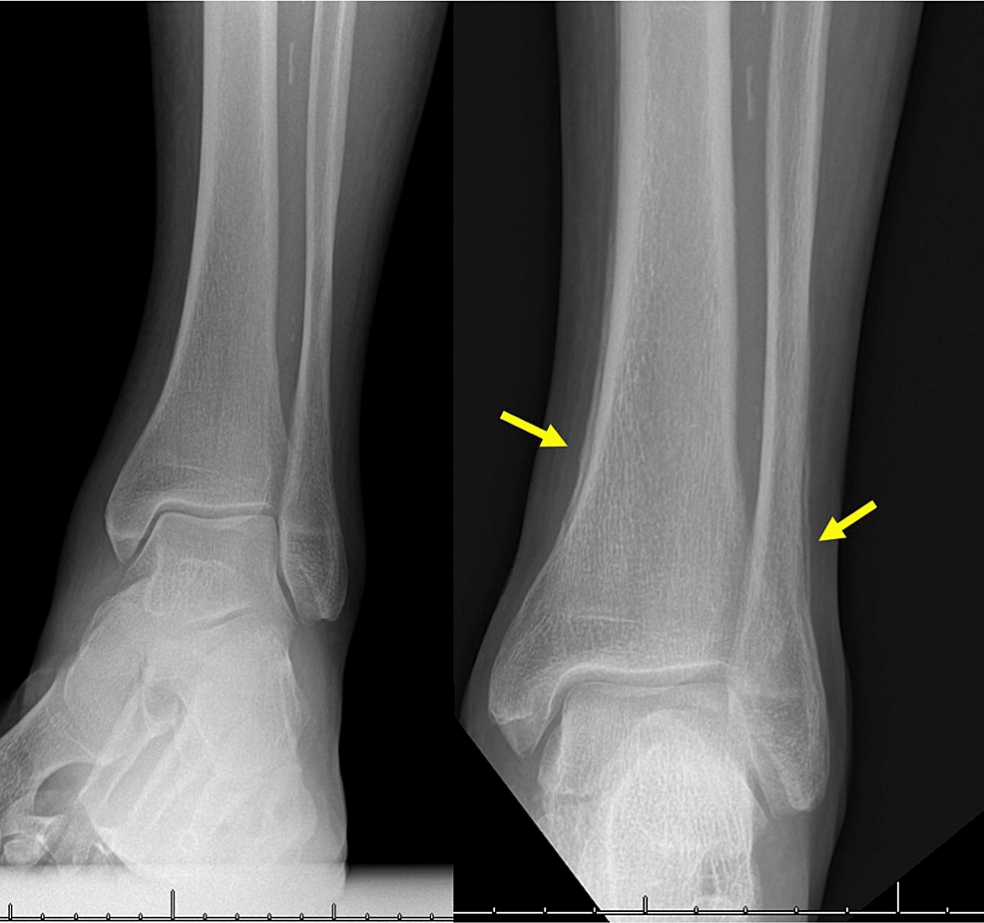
Radiological osteoarticular manifestations of the Pierre-Marie Bamberger syndrome X-ray of the left ankle in five-month intervals demonstrating the appearance of hypertrophic osteoarthropathy (arrows) and periostosis of the tibia and fibula
